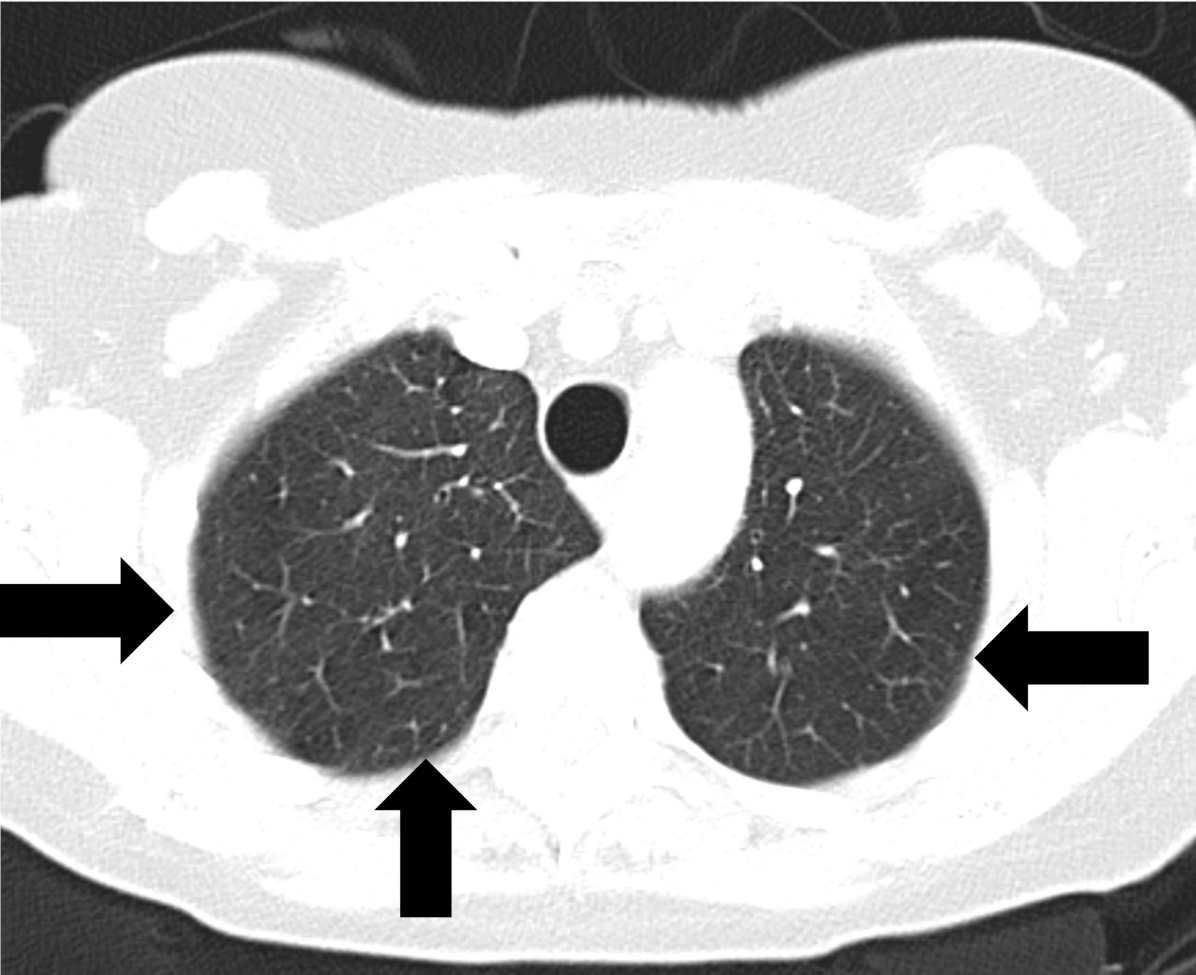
Thoracic CT scan showing the disappearance of all consolidation areas (second relapse). Absence of previously present parenchymal lesions (black arrows).
